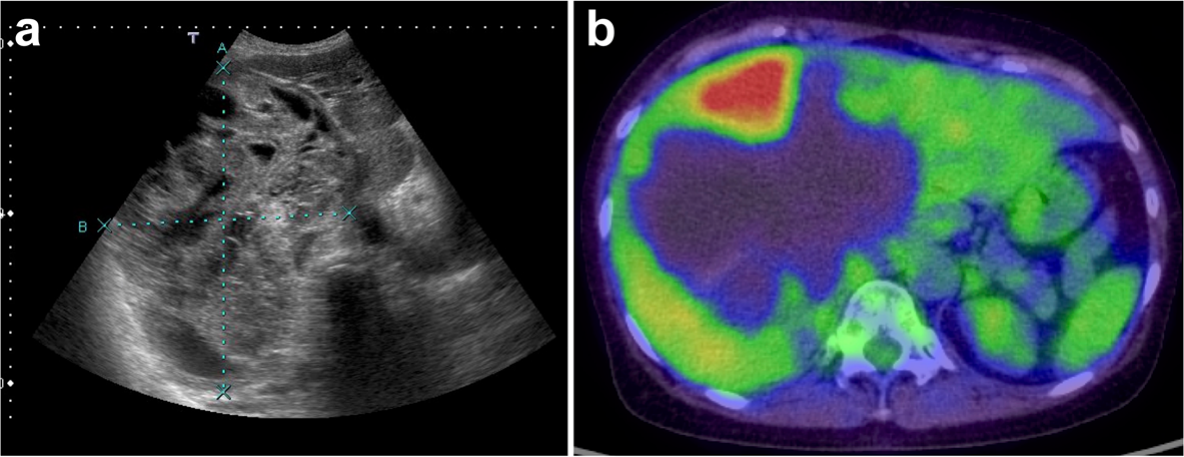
Abdominal US revealed a honeycomb pattern in the tumor, with solid components (a). PET-CT showed the uptake of FDG in the solid components of the cyst (b)
